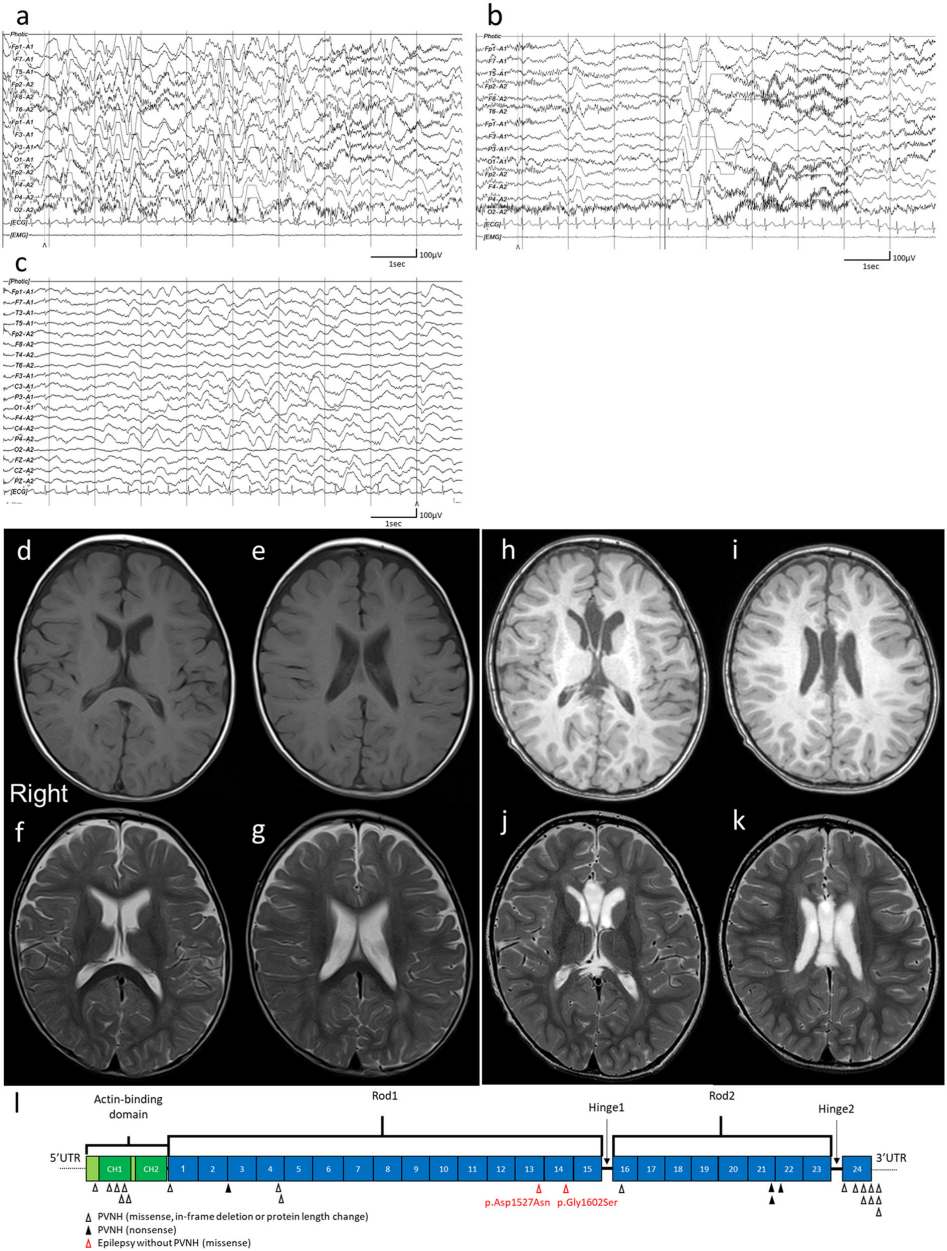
Clinical information of the patient and structure of filamin A protein. a Electroencephalography (EEG) results of the patient showing hypsarrhythmia during wakefulness at 9 months of age. b Ictal EEG results showing cluster spasms with head drop at 9 months of age. c EEG results at 11 months of age and after adrenocorticotropic hormone therapy. Sporadic slow waves were observed in the bitemporal regions. d, e Brain MRI T1-weighted and f, g T2-weighted images of the patient at the age of 15 months. Three pediatric neurologists independently confirmed the absence of periventricular nodular heterotopia. h, i Brain MRI T1-weighted and j, k T2-weighted images of the patient at the age of 29 months. l Filamin A protein with functional domains and FLNA variants found in males. Functional domains consist of the actin-binding domain containing two calponin homology (CH) domains and 24 Ig domains. Pathogenic variants detected in males are shown as triangles below Filamin A. FLNA variants in PVNH1 or in epilepsy without PVNH are shown as black or red triangles. Filled or open triangles indicate nonsense or missense/in-flame changes.
